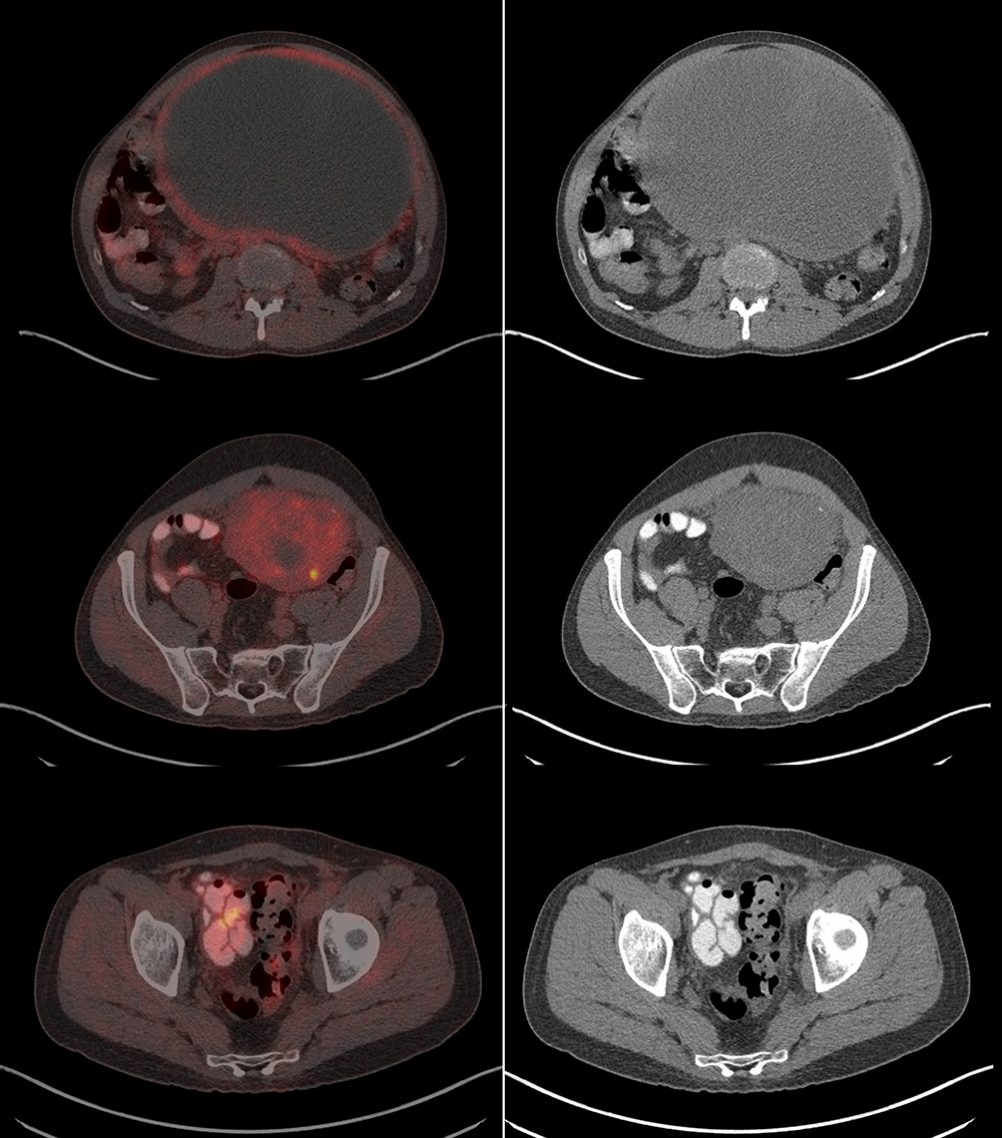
Post-radiation therapy positron emission tomography/CT fusion (left) and CT images (right) showing treatment response in the abdominal and peritoneal masses.
